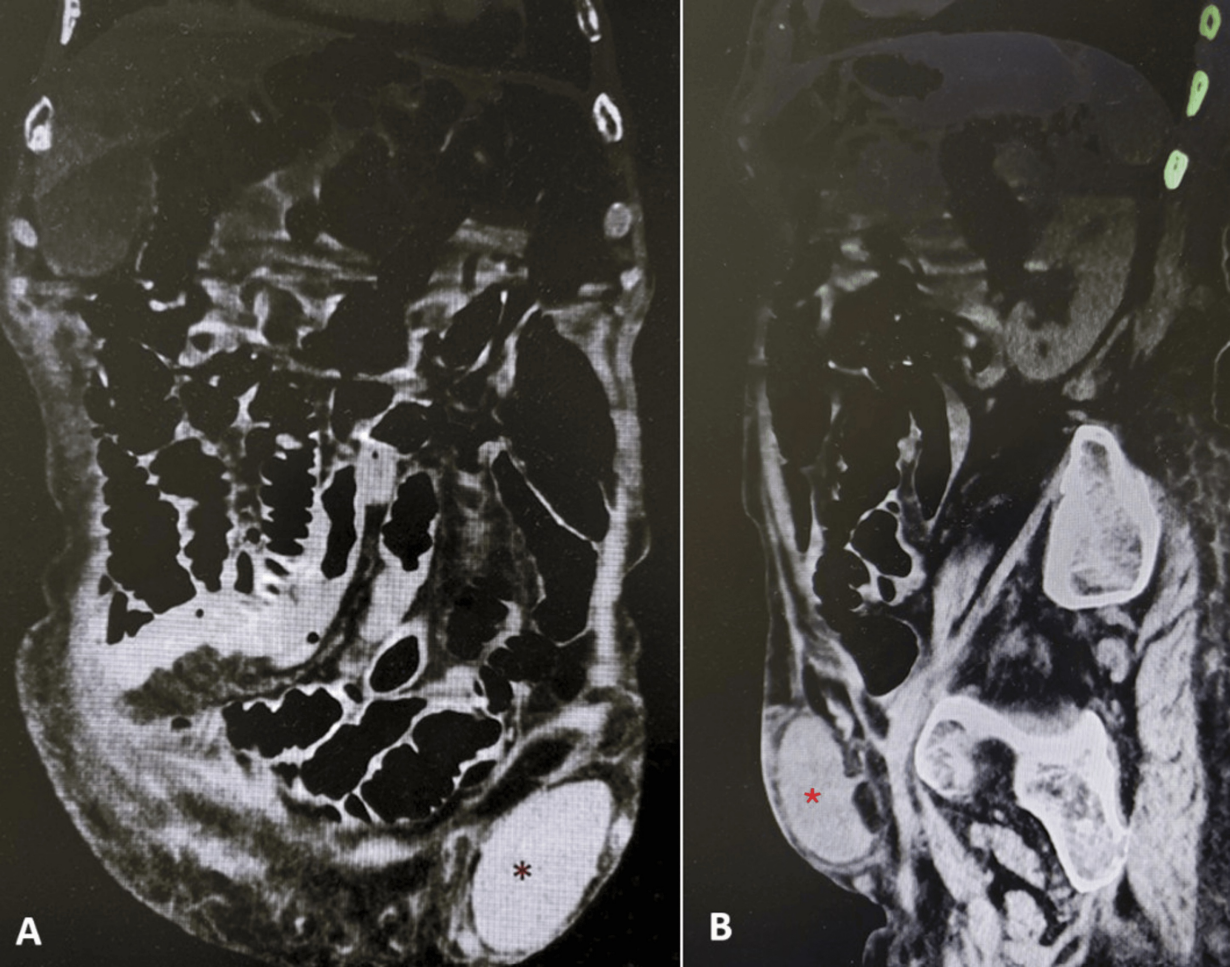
CT coronal (A) and sagittal (B) projections, showing a cystic lesion in the left inguinal canal with no apparent communication with the abdominal cavity (dark red and bright red asterisks) CT: computed tomography
